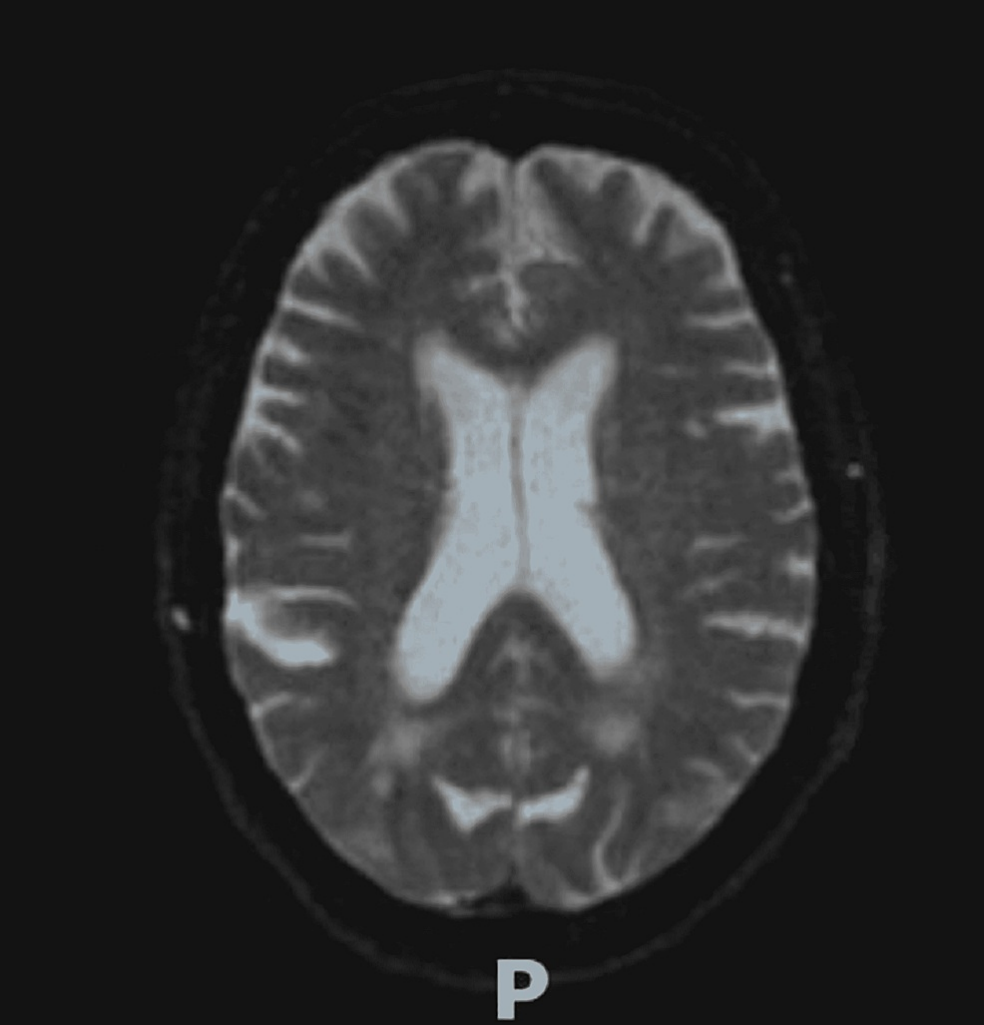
MRI with contrast showing previously seen white matter changes with PRES appear largely resolved PRES: posterior reversible encephalopathy syndrome
